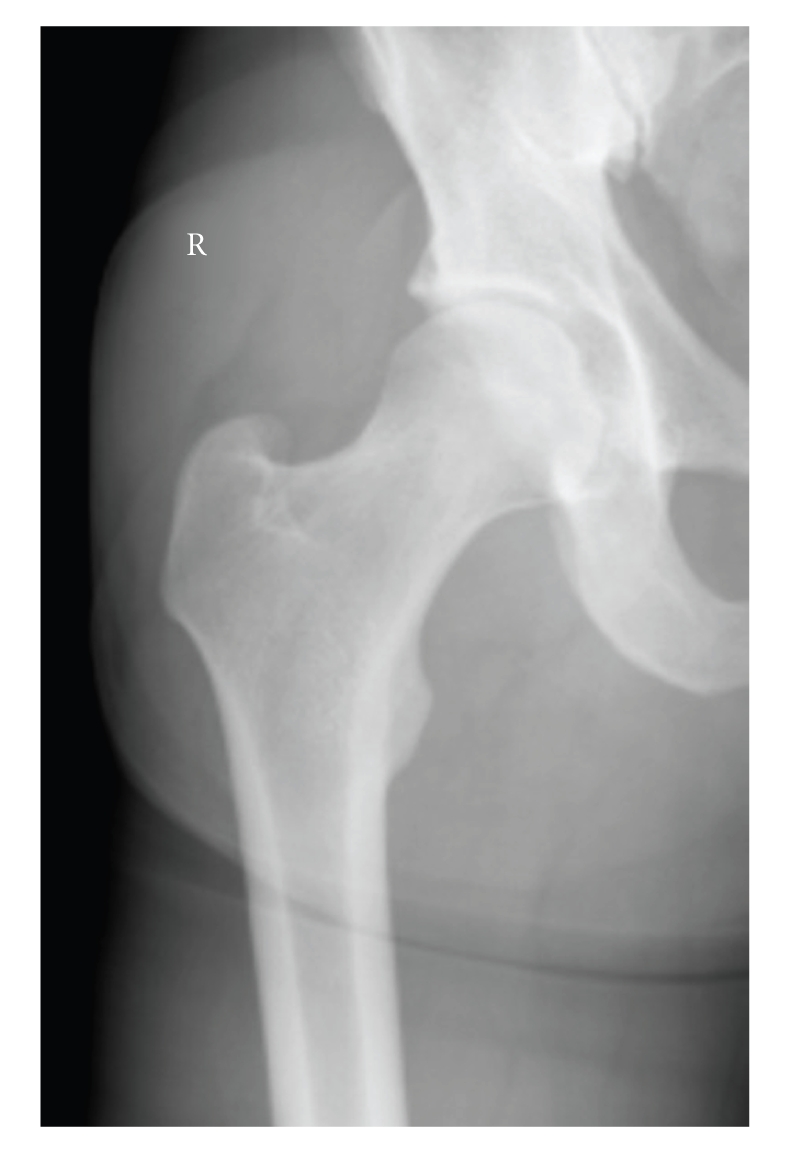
a/p X-ray one week after closed reduction shows a congruent hip joint and no fracture signs.
